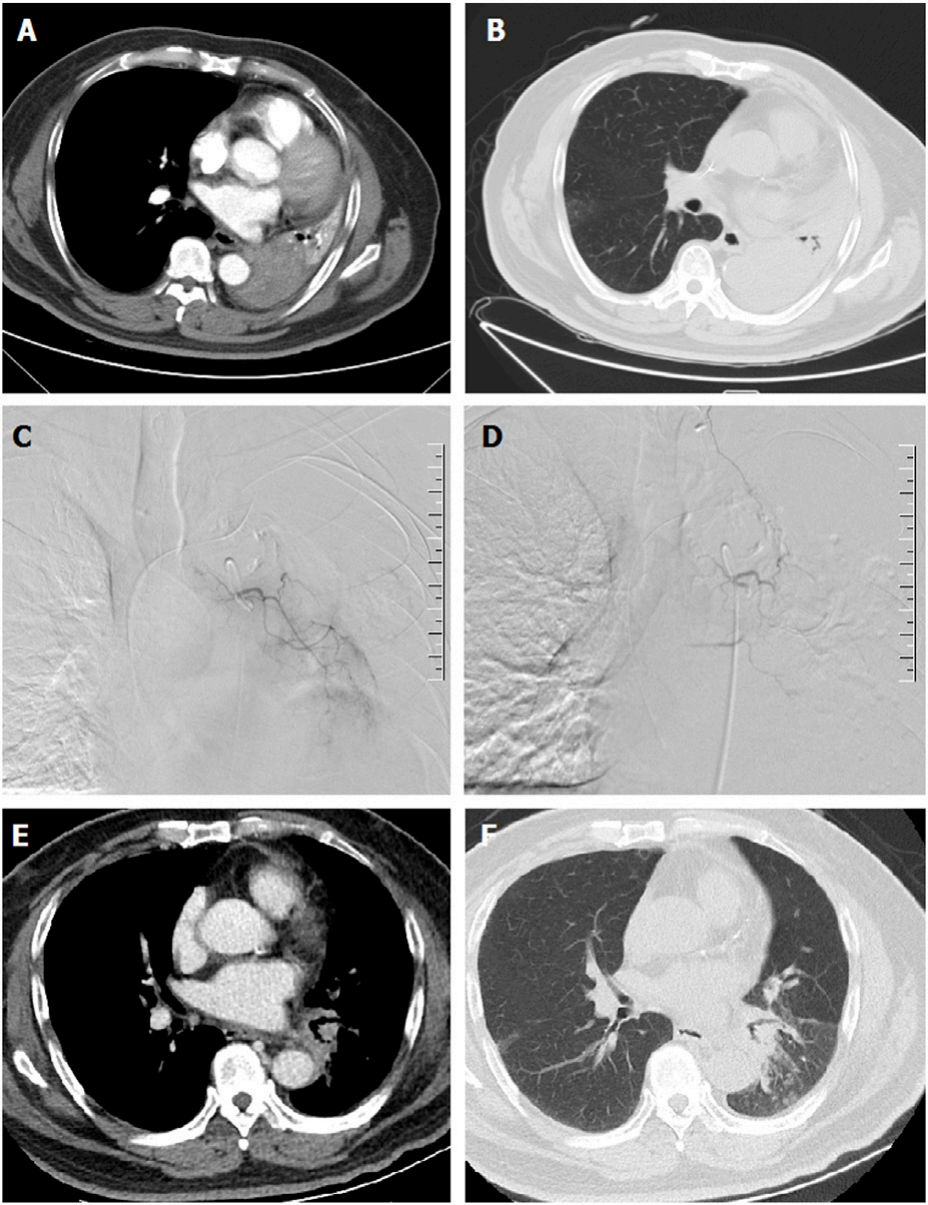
A 67-years male treated by CalliSpheres beads for advanced squamous cell carcinoma. (A,B) Computed tomography preoperative examination revealed a large tumor of the left lung with complete atelectasis. (C,D) The left bronchial artery was super-selectively incubated and embolized by drug-loaded beads. (E,F) The left lung tumor was found to shrink and the left lung atelectasis disappeared after 2 weeks’ follow-up.
